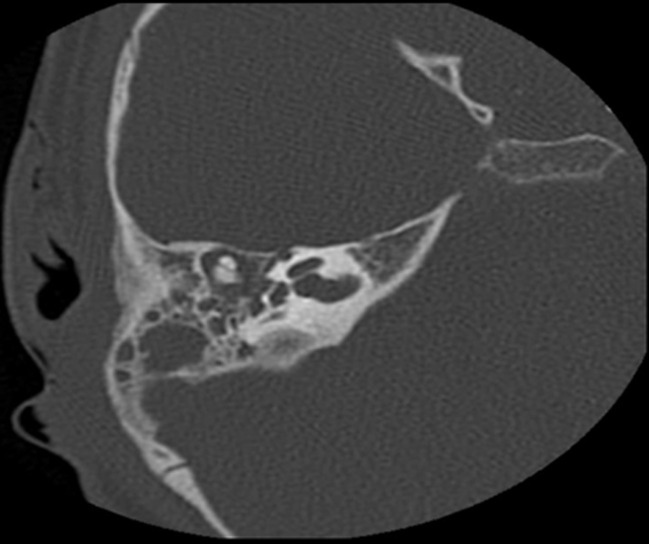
computed tomography scan of the craniofacial region showing tissue infiltration centered on the right retro-auricular region
